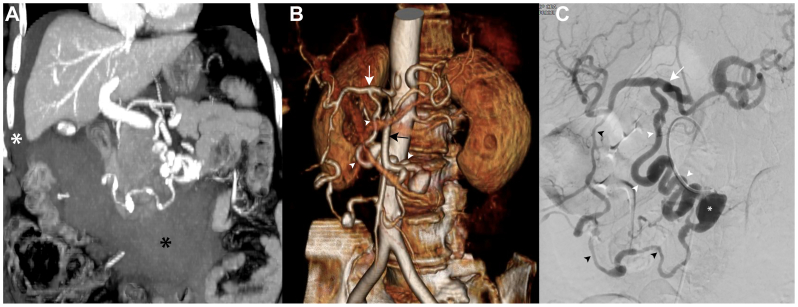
(A) Preoperative computed tomography angiography (2.5 mm thick; maximum intensity projection oblique coronal reconstruction) showing a massive retroperitoneal hemorrhage (*black asterisk*) and perihepatic hemoperitoneum (*white asterisk*). (B) Volume reconstruction depicting the ectatic and tortuous arc of Bühler (AOB) connecting the superior mesenteric artery (SMA) (*black arrow*) and the celiac trunk (*white arrow*). The AOB (*white arrowheads*) runs behind SMA owing to his retropancreatic position. (C) Digital subtraction angiography performed from SMA showing the double connection between the celiac axis (*white arrow*) and SMA (point of injection) by the tortuous AOB (*white arrowheads*) and the pancreaticoduodenal arcade (*black arrowheads*) characterized by irregular diameter. The angiogram shows a 22-mm true aneurysm (*white asterisk*) at the inferior origin of the pancreaticoduodenal arcade.
